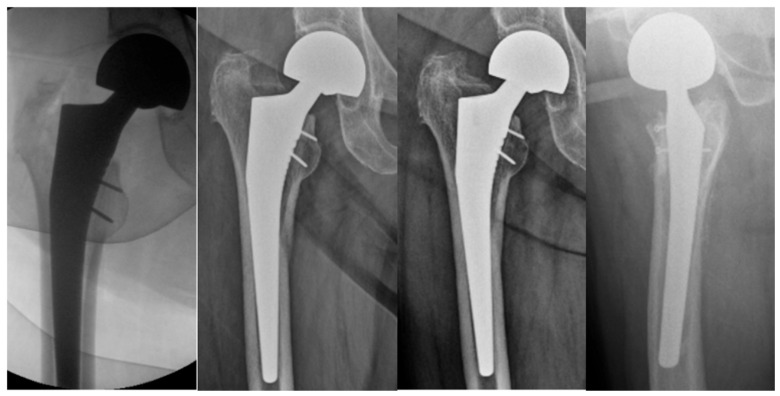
Intraoperative image showing intraoperative fluoroscopy of a periprosthetic proximal femoral fracture at the calcar level, fixed with two 2.7 mm screws, along with 2- and 5-month (AP and axial view) postoperative x-rays showing subsidence and then healing of the fracture with 1 cm of shortening.
